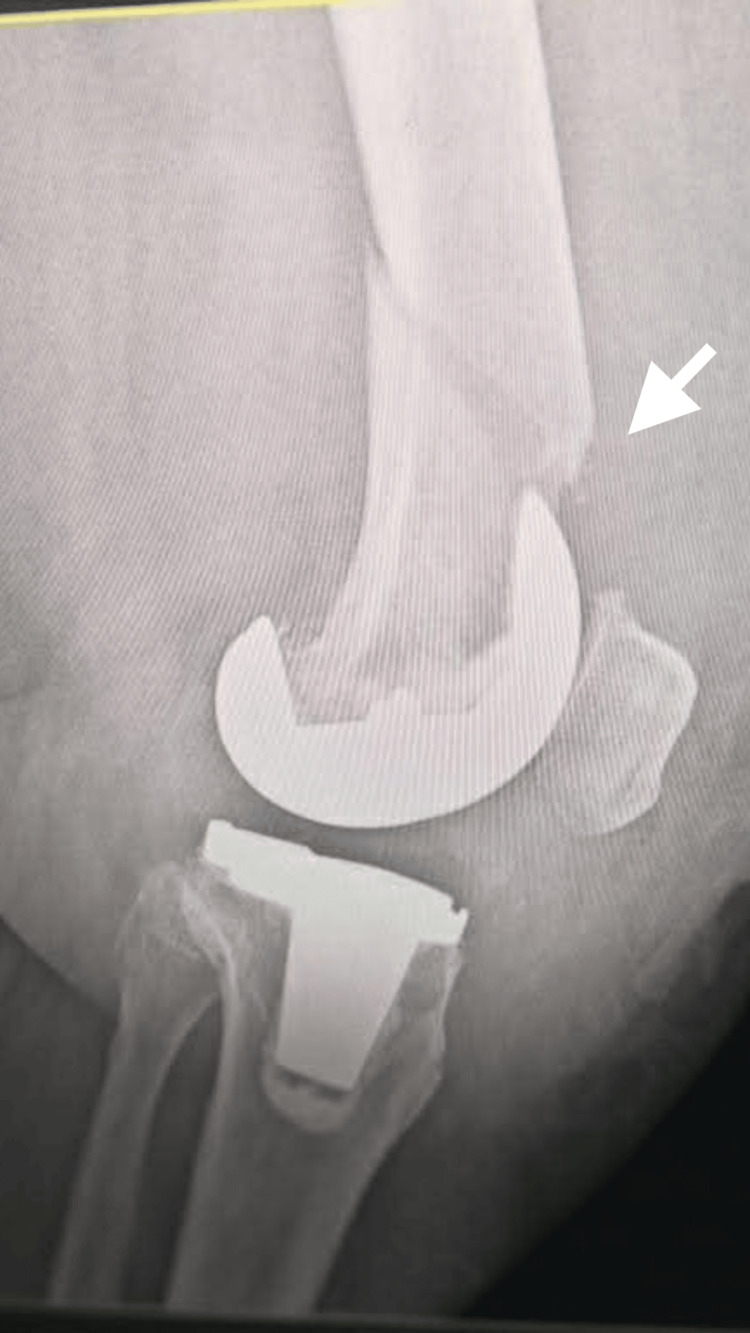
Lateral radiograph of the left knee Lateral radiograph of the left knee demonstrating a supracondylar periprosthetic distal femoral fracture above the femoral component following total knee arthroplasty. The arrow indicates the fracture line proximal to the femoral prosthesis.
